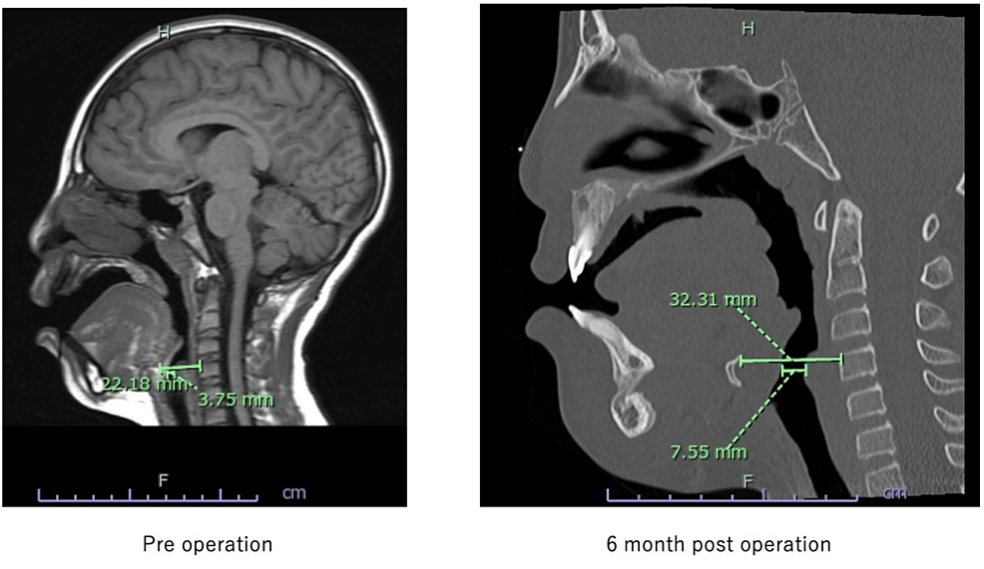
Pre- and postoperative sagittal X-ray views. Advancement of the hyoid bone and expansion of the airway are obvious.
